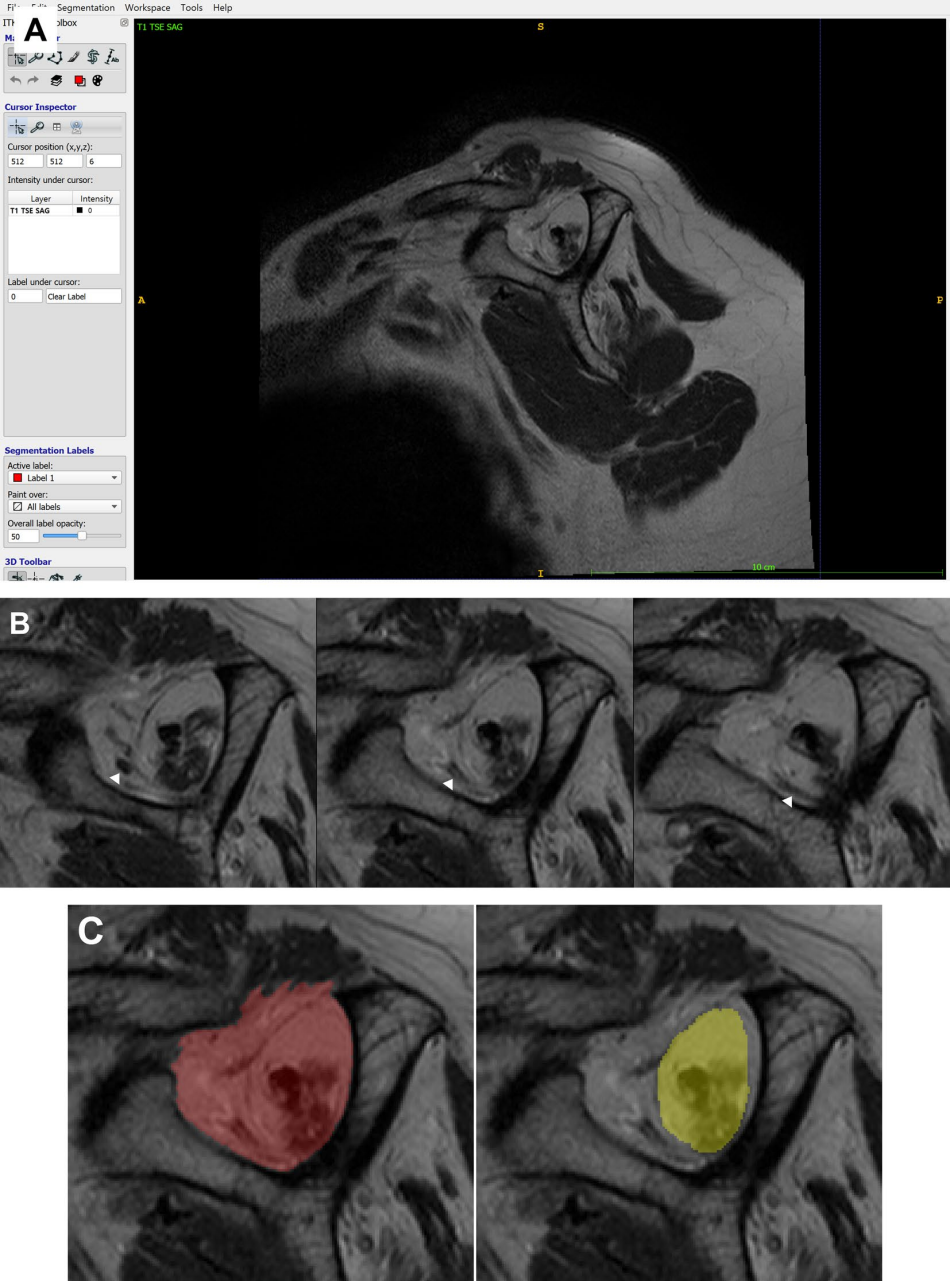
Annotation using ITK-SNAP software: (A) loading the MR image of oblique sagittal view on the ITK-SNAP; (B) detection of the scapular Y-view and identification of the margin of supraspinatus fossa and supraspinatus muscle. The arrowhead indicates the vessel around the supraspinatus muscle; (C) annotation of the supraspinatus fossa and supraspinatus muscle using the brush tool of ITK-SNAP.
